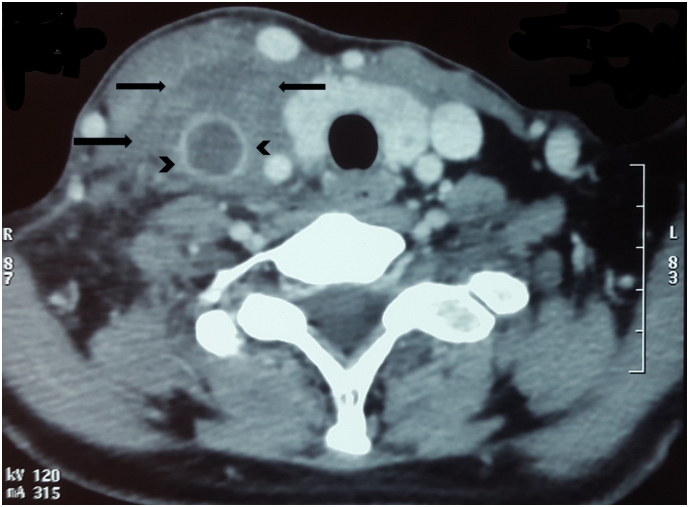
On axial contrast-enhanced computed tomography scan an abnormal low attenuation within the para-pharyngeal space and along the carotid space indicates.
